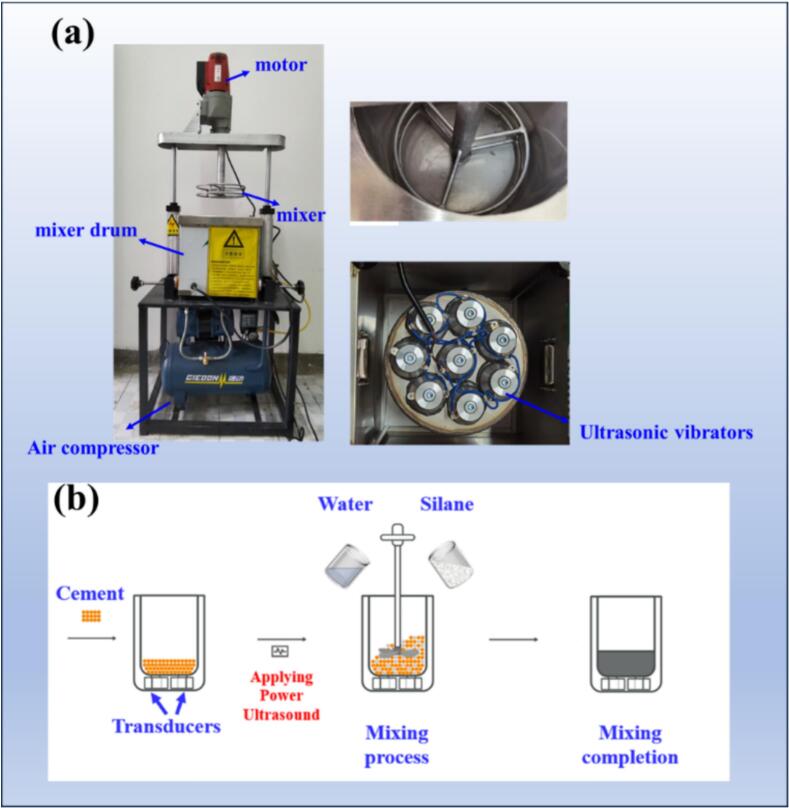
Ultrasound equipment used for the test.
